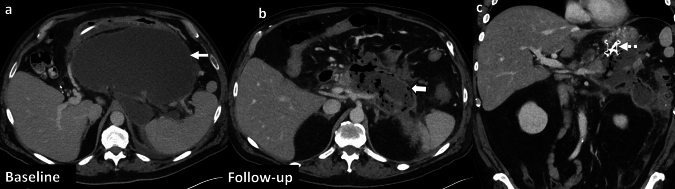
Air within necrotizing pancreatitis due to iatrogenic interventions. A 45-year-old with alcohol induced necrotizing pancreatitis. Baseline CT image (a) shows large walled-off necrosis (WON) replacing the pancreas (white arrow, a). This was treated with endoscopic cyst-gastrostomy using an Axios® stent. Follow-up CT images show decreased size of the WON with new post-procedural air (white block arrow, b) and the cyst-gastrotomy stent (dotted white arrow, c)
